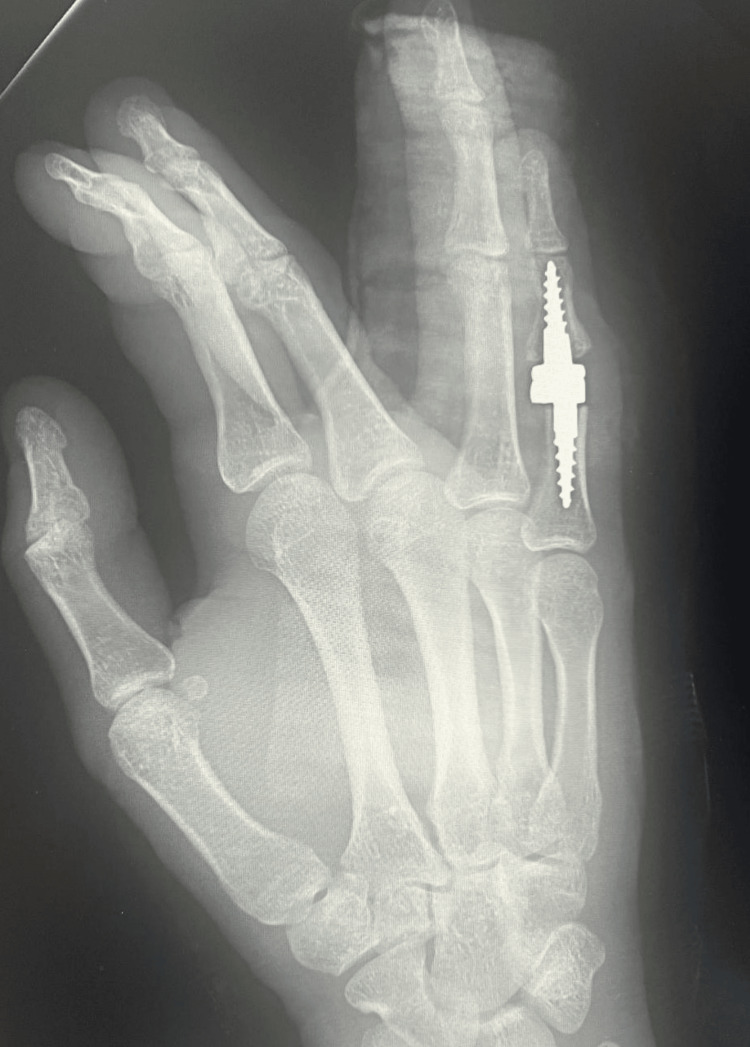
X-ray of the right hand in the lateral projection on the first day after surgery
